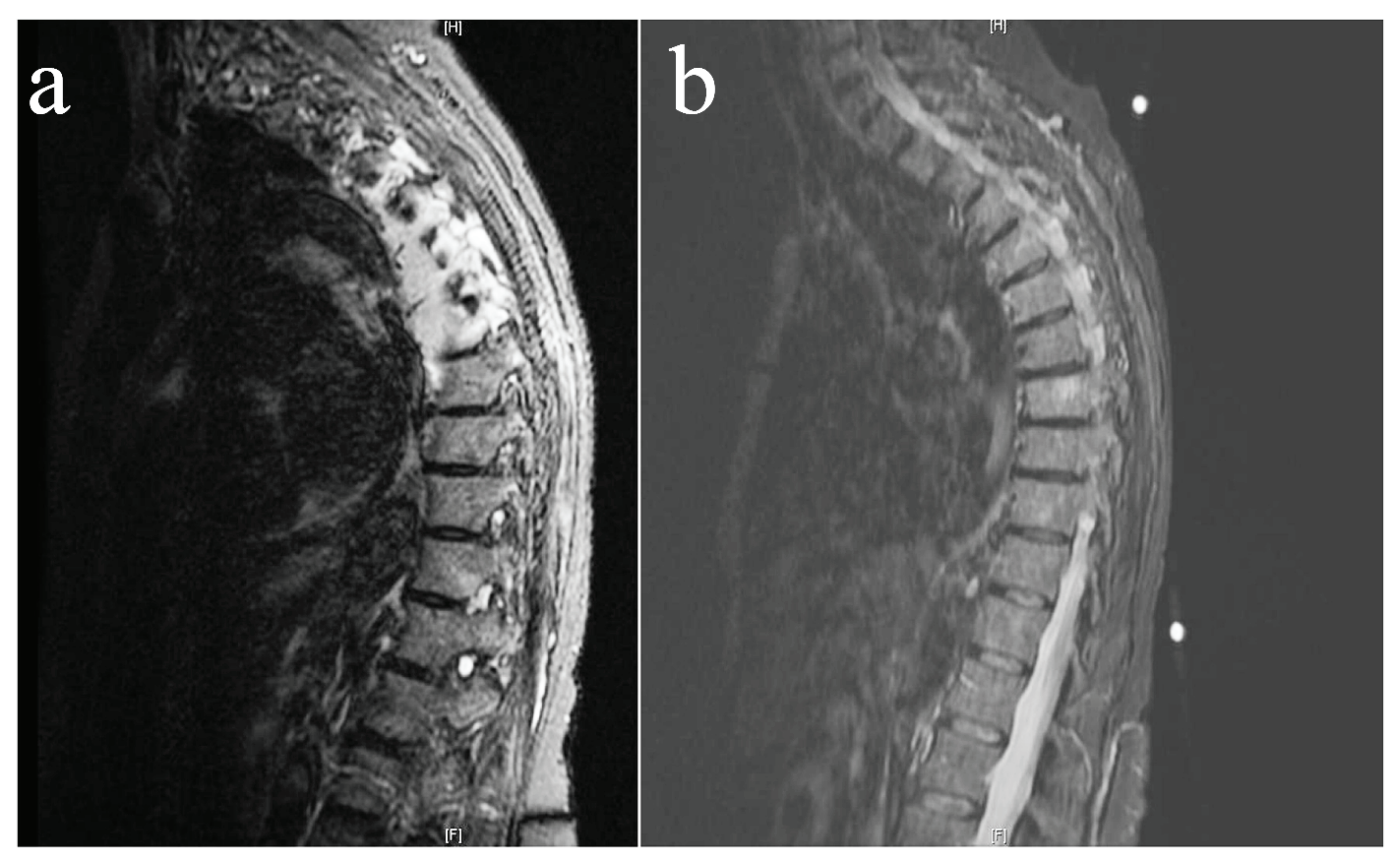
Pretreatment (a) and post-treatment (b) T2-weighted MRI showing a near complete resolution of the paraspinal metastatic disease and epidural soft tissue extension, with no evidence of cord compression after three cycles of R-CHOP and intra-thecal methotrexate.
